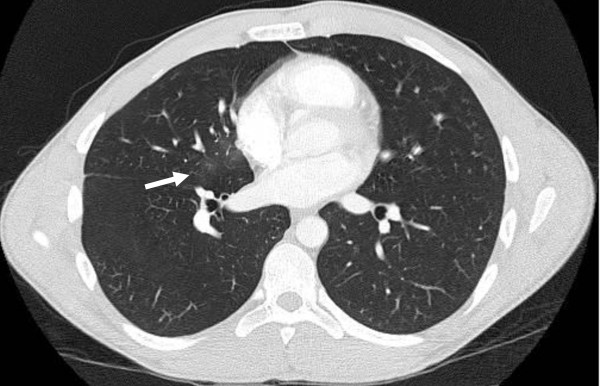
Resolving pulmonary contusion. CT of the chest one week after injury showing near-complete resolution of the pulmonary contusion.
